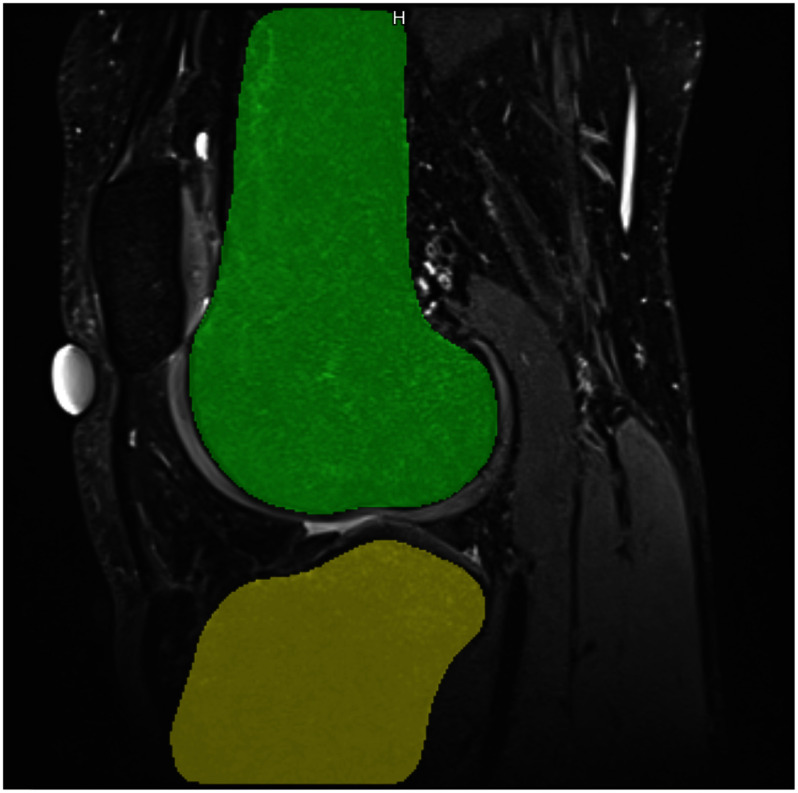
Sagittal view of the tibia and femur segmentation (in color) superimposed with the original MRI scan of a healthy human knee.
